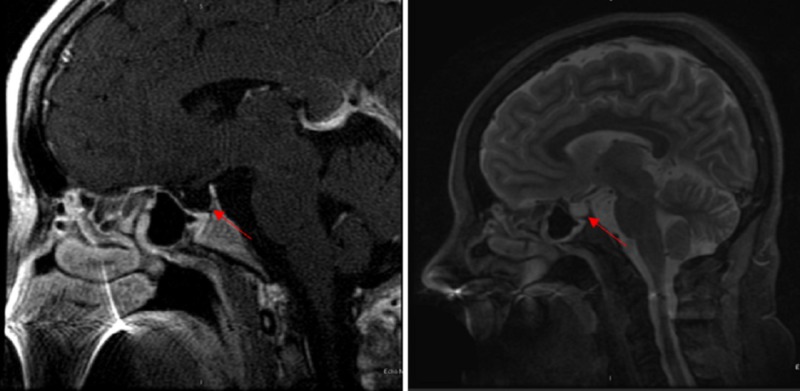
Magnetic resonance imaging (MRI) of the brain showing an empty sella
